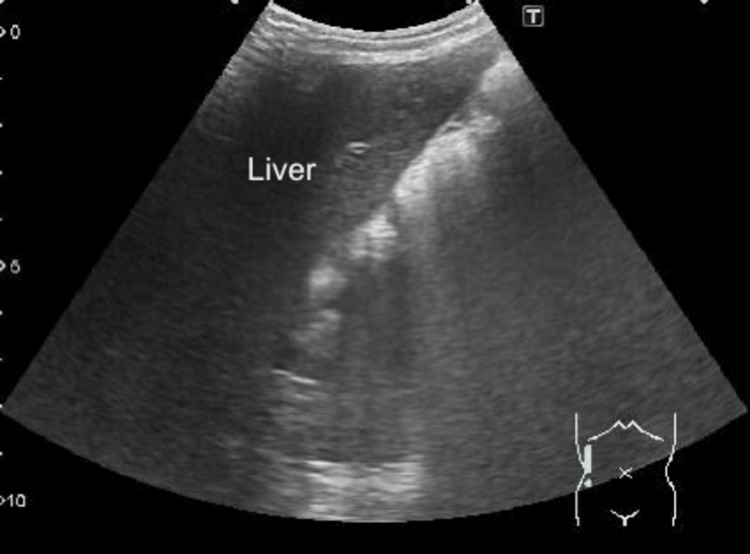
Abdominal POCUS finding (right abdominal short axis view) Ultrasonography demonstrated neither target nor pseudo-kidney signs in the ascending, transverse, and descending colons. Furthermore, no right kidney or free peritoneal fluid adjacent to the liver was detected in the right peritoneal cavity. POCUS: Point-of-care ultrasound.
